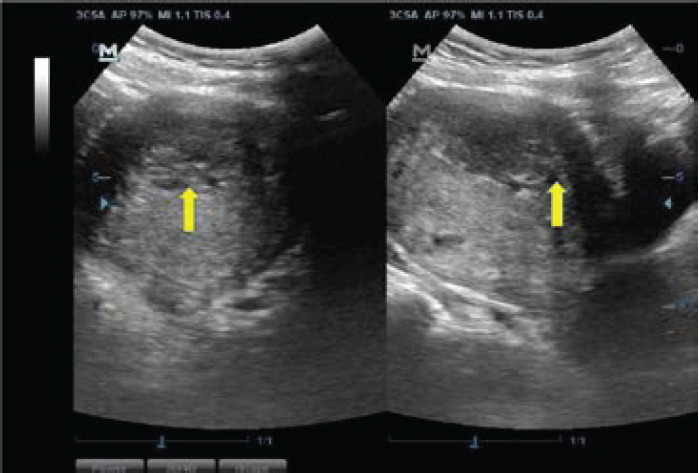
An ultrasound scan of the uterus showing a submucosal predominantly hypoechoic anterior myoma
